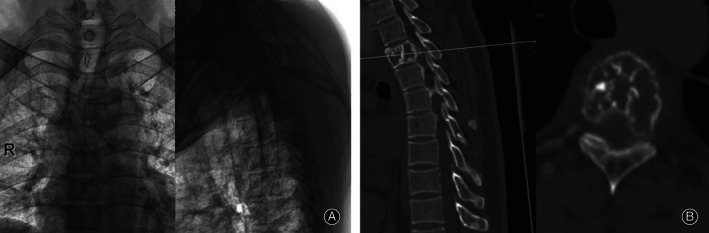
The patient had unbearable back pain and came to the hospital for treatment: (A) X‐ray showed no collapse of the T_4_ vertebral body shape and (B) the CT showed severe bone destruction.
